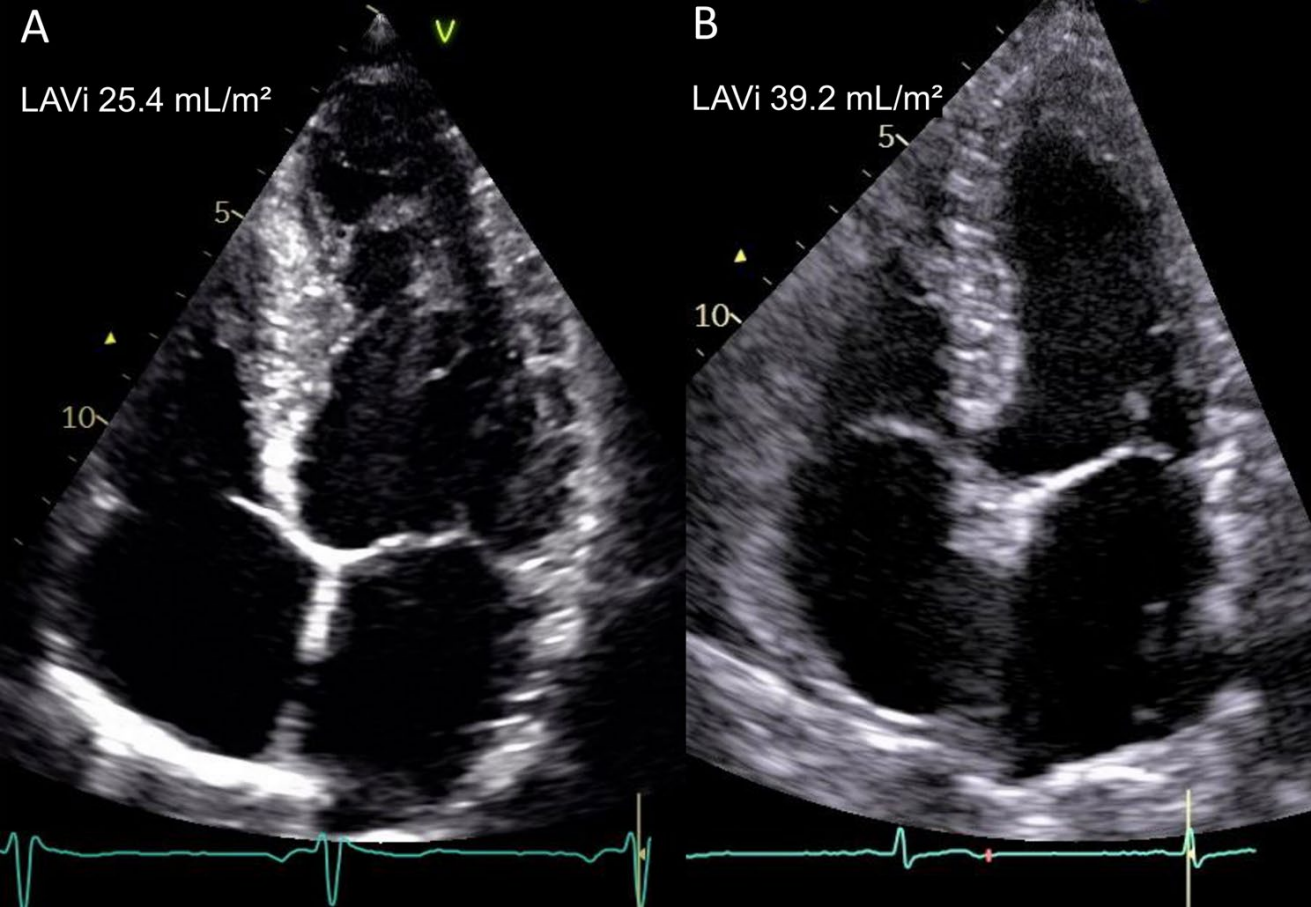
Echocardiographic images of patients with AMI. The image to the left (A) shows a patient with normal left atrial volume who survived the study period whereas the image to the right (B) shows a patient with dilated left atrial volume who died
